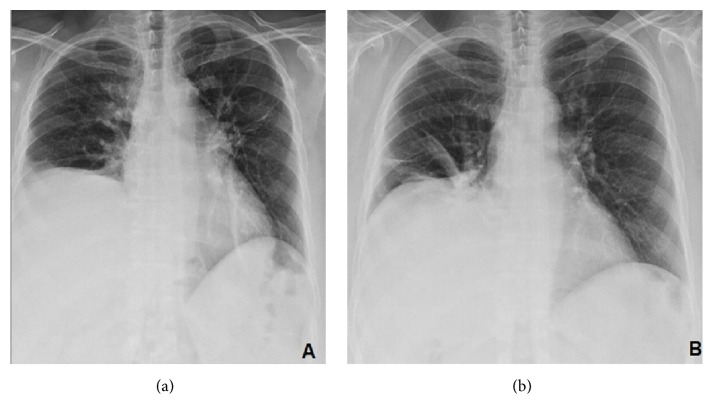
(a) Chest X-ray of the day after the radiofrequency catheter ablation (RFCA) in deep inspiration showing right hemidiaphragm paralyzed. (b) Chest X-ray 6 months after acute event.
